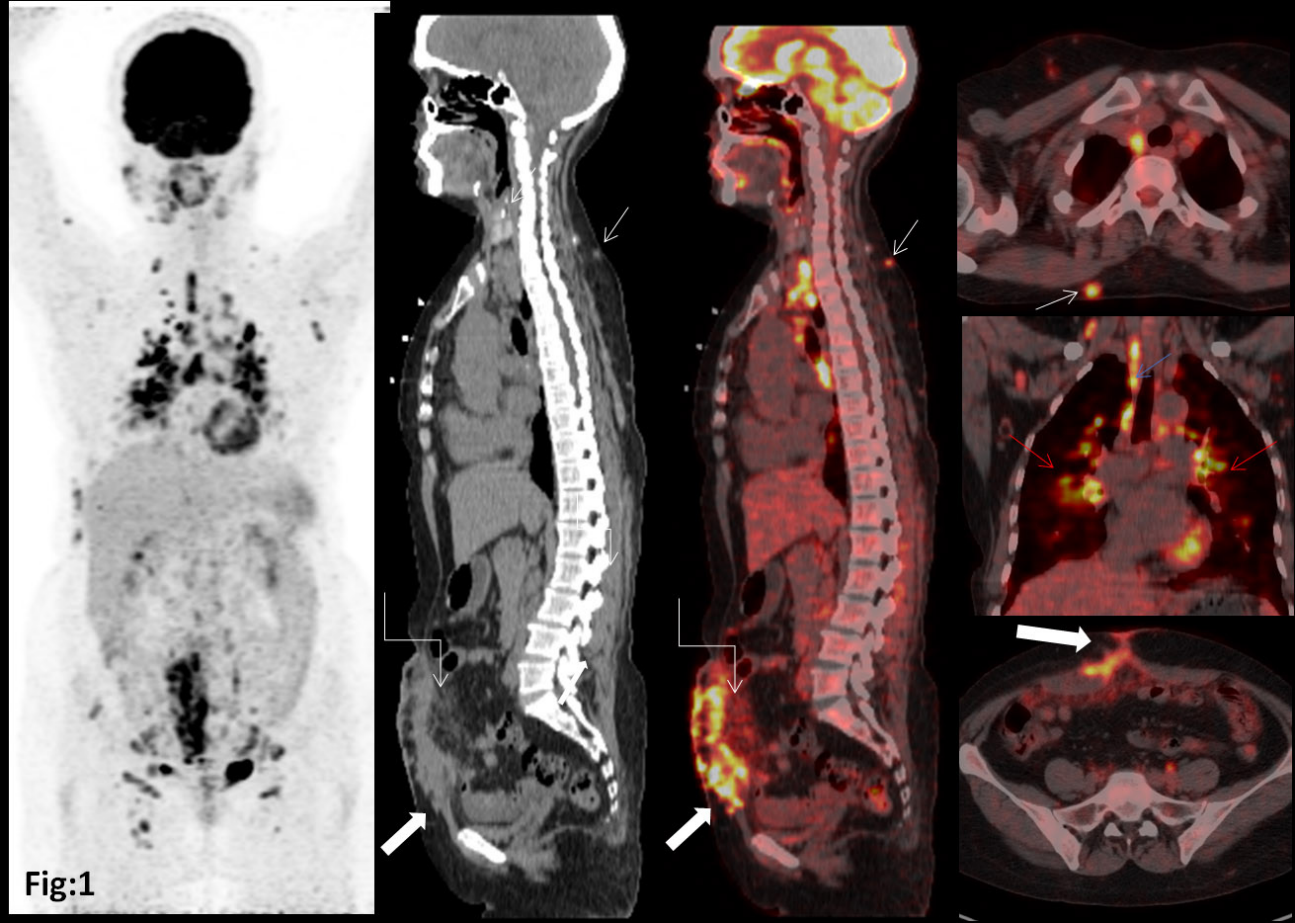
^18^F-FDG PET/CT show increased FDG uptake in multiple subcutaneous nodules (small white arrow), mediastinal nodes (blue arrow), and bilateral lung parenchymal involvement and anterior abdominal wall scar (thick white arrow) with the involvement of the omentum (curved white arrow)
